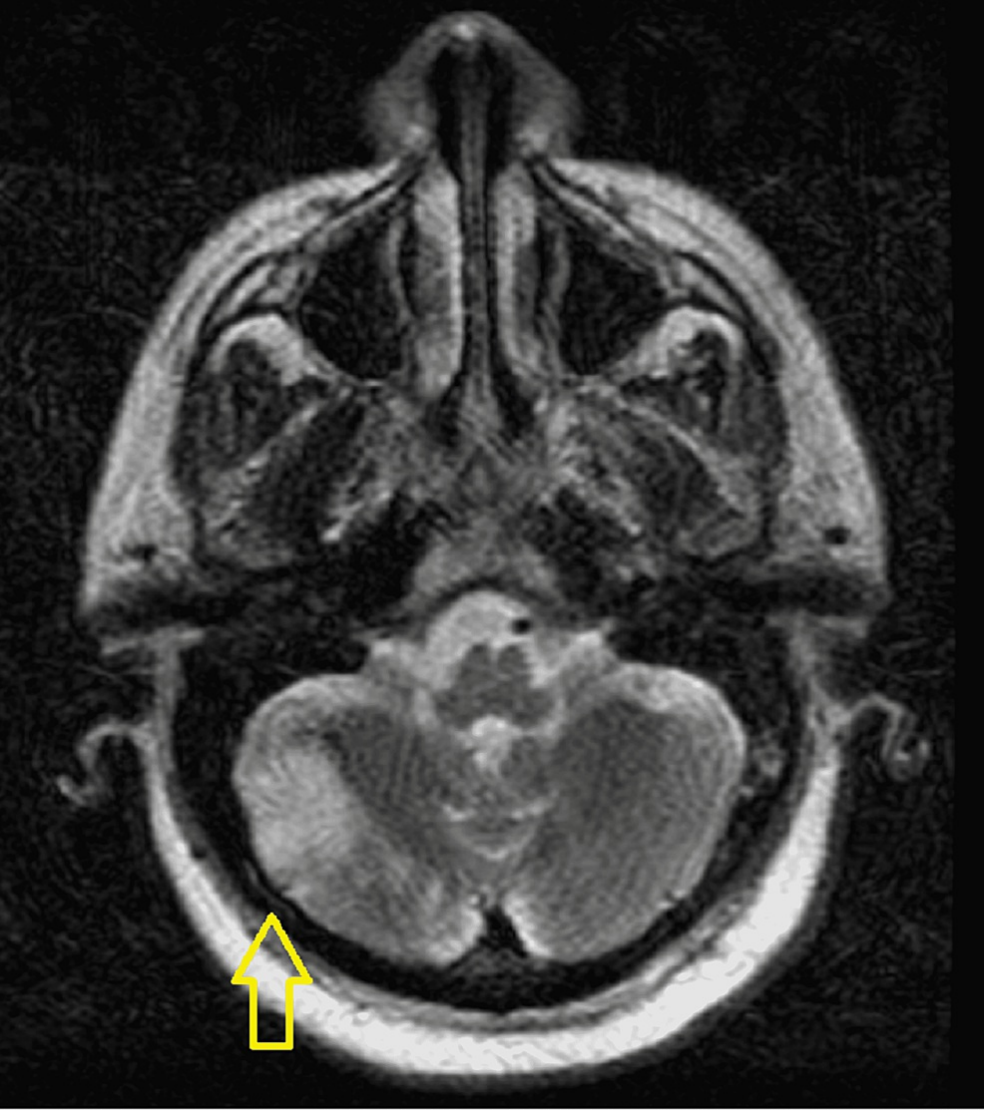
MRI of the brain showing right-sided cerebellar infarct.
